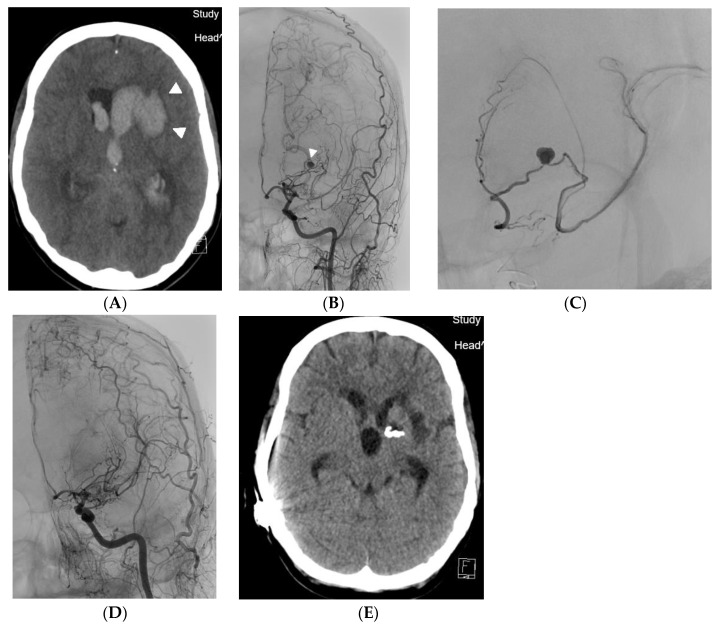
(A) Case 1. Native CT scan showing an intraparenchymal hematoma (pair of arrowheads) that has spread into the lateral ventricle. (B) Case 1. The hemorrhage was caused by an aneurysm on the lenticulostriate collateral (arrowhead), which bridges a narrow stenosis of M1 section of the middle cerebral artery. (C) Case 1. Selective angiography of the lenticulostriate artery with an aneurysm filling distally the M2 branch of the middle cerebral artery. (D) Case 1. Final angiogram after embolisation, where the aneurysm does not fill. DSA after 10 months confirmed permanent closure of the bleeding aneurysm. (E) Case 1. CT scan demonstrating placement of the acrylic embolisation mixture cast.
